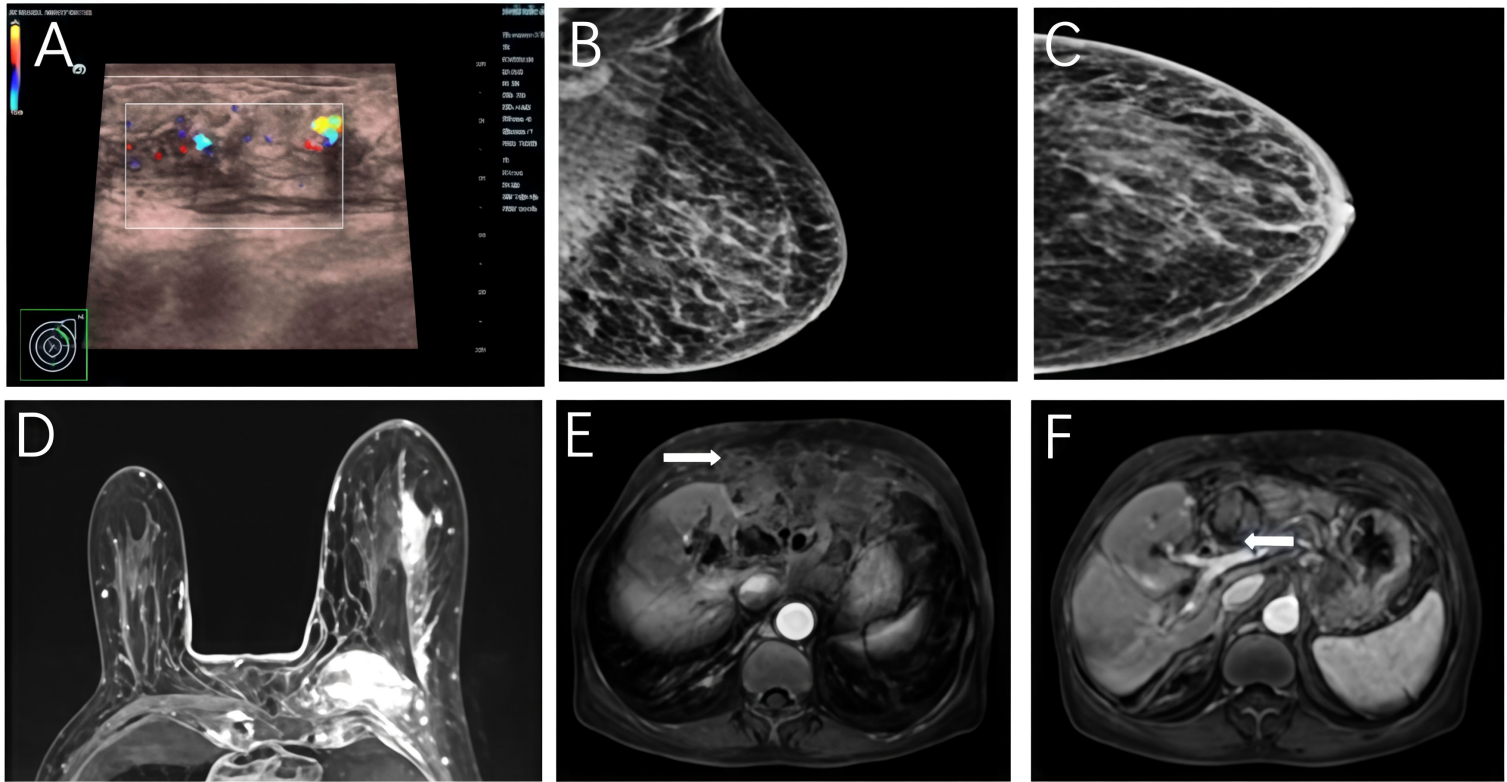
Imaging findings of the patient. (A), Breast ultrasound reveals an irregular hypoechoic lesion with increased internal vascularity. (B, C), Mammography (B), mediolateral oblique view; (C), craniocaudal view) shows diffuse architectural distortion and increased parenchymal density in the left breast with ipsilateral axillary lymph node enlargement. (D), Contrast-enhanced breast MRI demonstrates extensive non-mass enhancement in the upper outer quadrant of the left breast. (E, F), Abdominal contrast-enhanced MRI reveals an irregular hepatic mass with intrahepatic bile duct dilatation (E) and abutment of the mass to the chest wall and pericardium, suggesting local invasion (F).
